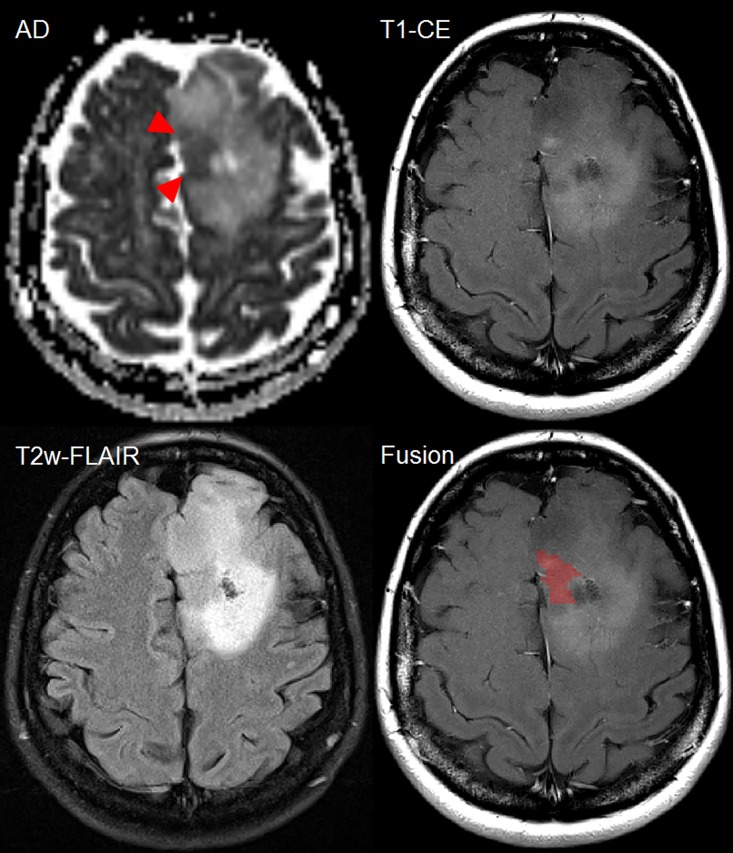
Female patient (44y) with malignant transformation of a primary resected oligoastrocytoma WHO II into anaplastic oligoastrocytoma III in the left frontal lobe demonstrating heterogeneity within the patchy contrast-enhancing region. Increased diffusion restriction (red triangles) is noted at the same timepoint as new CE. Within the patchy area of CE, the spatial position of hypercellularity is visualized as red cluster (last image). Comparing T2w-FLAIR and axial diffusivity, the hypercellularity is found to be located in a T2w-hypointense region with a punctual CE focus. However, large proportions of patchy CE do not match with the diffusion restriction. Such information is important to consider for guidance of stereotactic biopsy and focal treatment planning.
